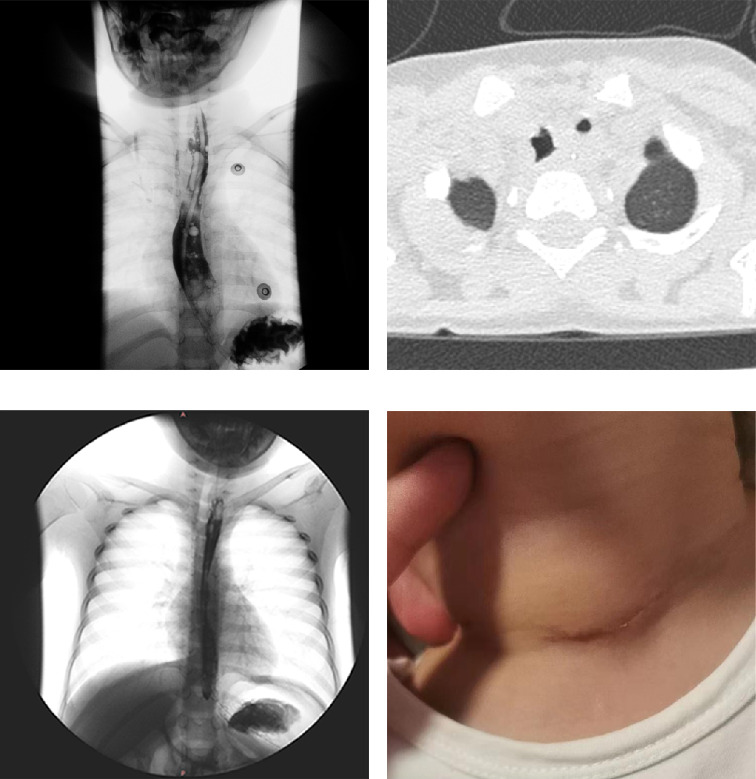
(a) A possible fistula between the trachea and esophagus at the T1-T2 level under esophageal iodine contrast imaging. (b) Re-evaluation of the chest CT scan demonstrates significant improvement in the pulmonary inflammation, no foreign bodies visualized within the trachea and esophagus, and the perforation has healed significantly compared to before. (c) Esophagography shows significant improvement in the tracheoesophageal fistula, with most of it healed compared to before. (d) The well-healed wound at the tracheostomy site in the pediatric patient.
